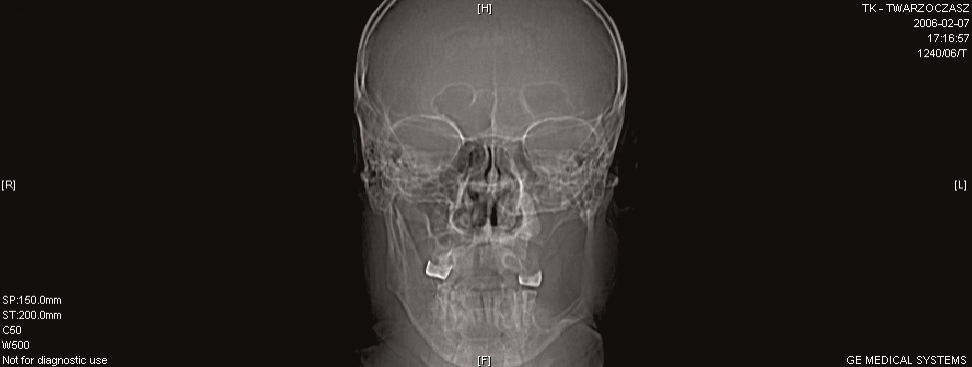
Cranial X-ray. The figure demonstrates a well-delineated osseous defect spreading throughout the left mandibular ramus, infiltrating and destroying the structures of the pterygopalatine fossa.
